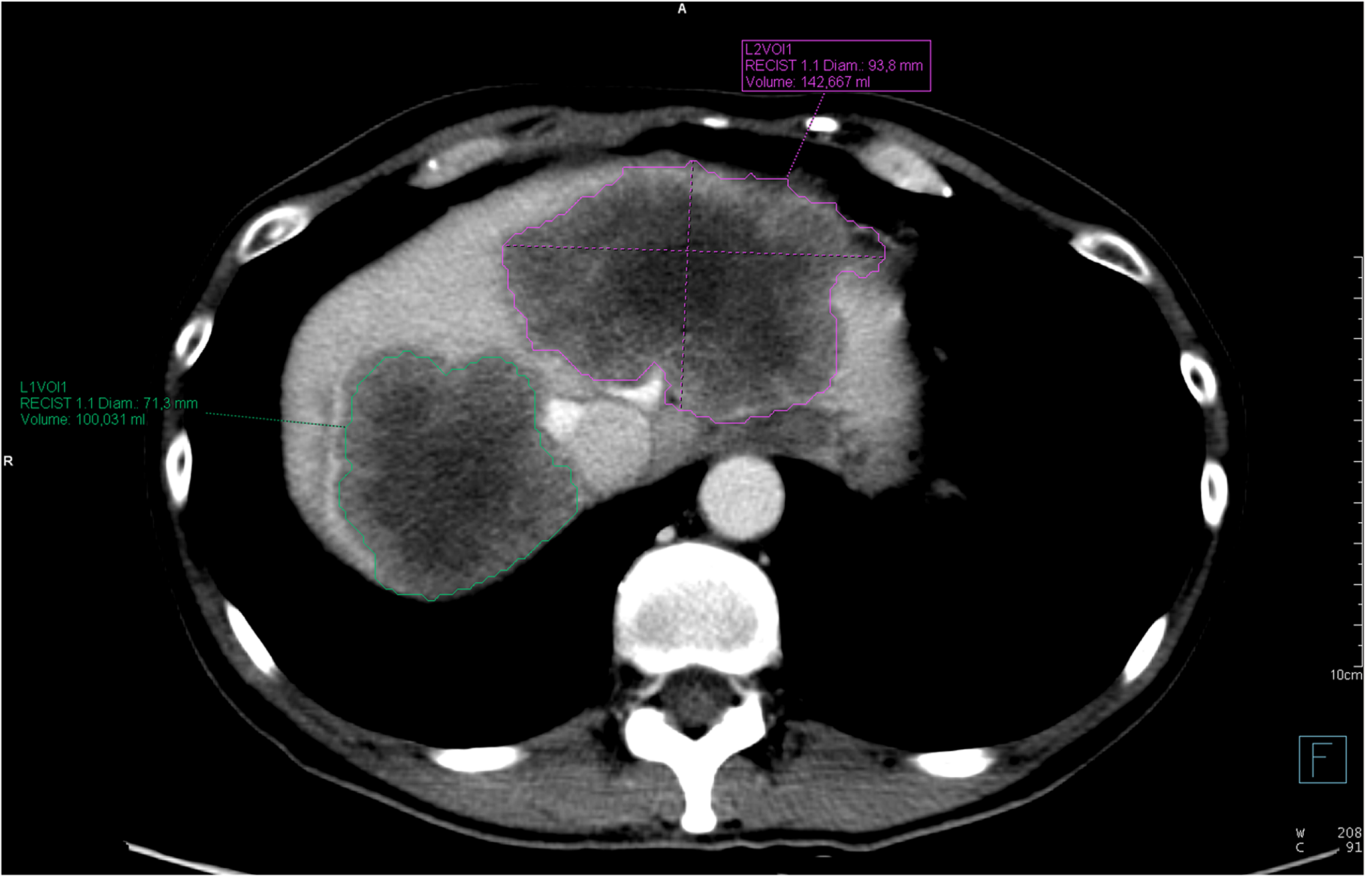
Example of volumetric segmentation. Computed tomography scan of a 52-year-old female at baseline, after segmentation of two large liver lesions as proposed by the software. Afterwards, minor manual corrections of the contours were necessary. One smaller lesion in segment V is not shown. Receiving FOLFIRI plus bevacizumab, the patient had a diametric early tumor shrinkage (ETS) of 26.3% and a volumetric ETS of 65.7% in the first restaging. She had progressive disease after 7.8 months and died after 21.5 months. Abbreviations: ETS, early tumor shrinkage
